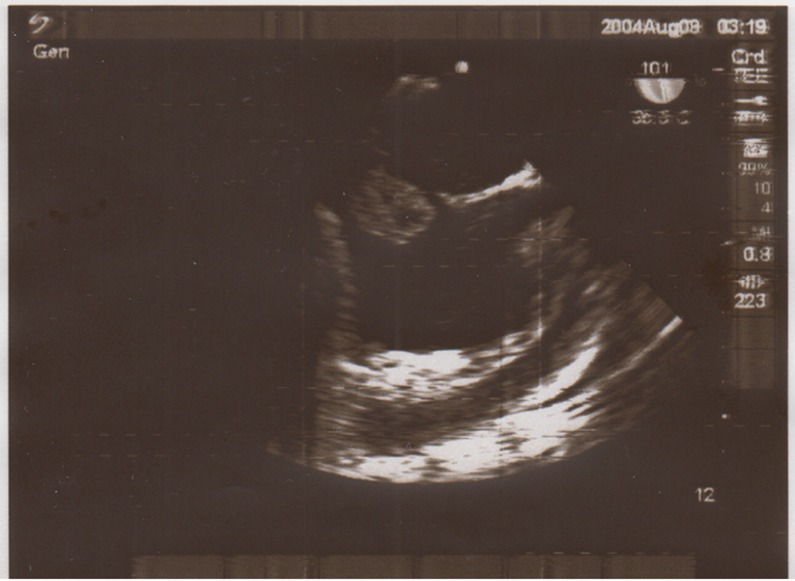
Transthoracic echocardiography M Mode view revealed a large clot in the entrance of inferior vena cava to the right atrium.
